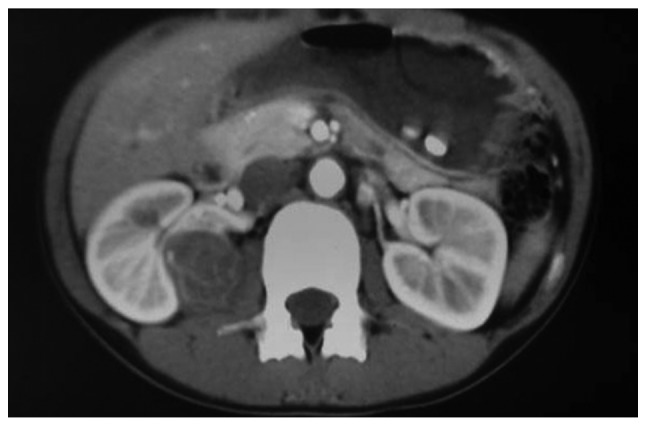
Computed tomography for the right renal hemangiopericytoma.
